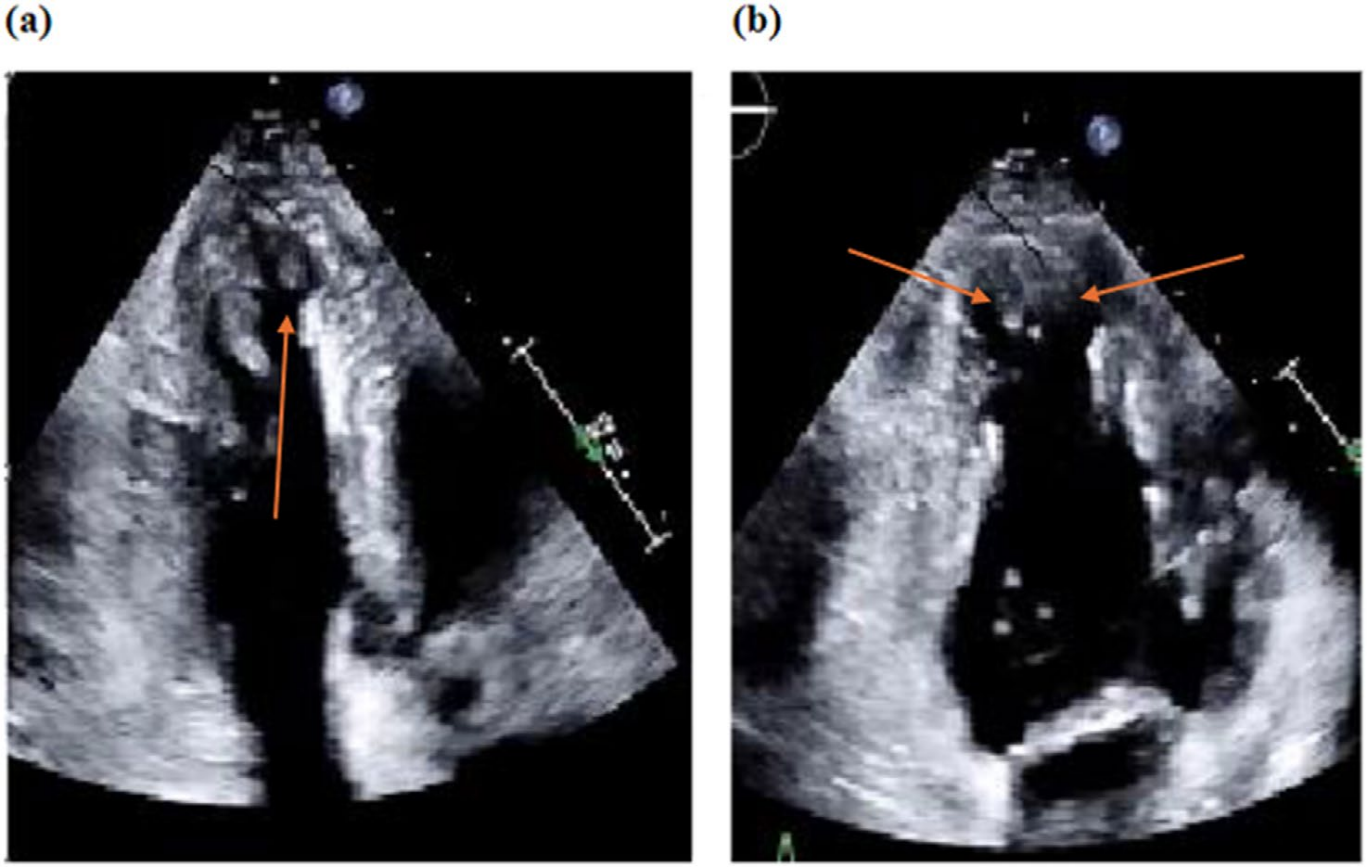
(a) 3 chamber (b) 2 chamber transthoracic echocardiogram showing apical trabeculations.
